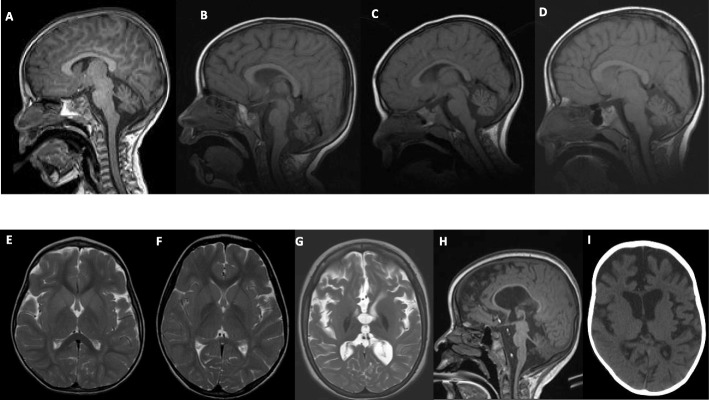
Radiographic Imaging Data for the Study Cohort. a-d: Representative MRI images for the affected individuals P14 (at age 3 years 2 months), P15 (at age 3 years 2 months), P19 (at age 4 years 3 months), and P20 (at age 4 years 7 months) showing cerebellar atrophy. e: MRI at 3 years and 2 months showing altered signal intensity in the globus pallidus of P15. f: MRI at 4 years and 3 months showing bilateral symmetrical subtle low T2 signals noted in the globus pallidus, substantia nigra, and dentate nuclei of P19. g: MRI at 6 years and 9 months showing bilateral globus pallidus low signal intensity likely representing iron deposition in P18. h: MRI at 6 years and 9 months showing brain, cerebellar and brainstem atrophic changes in P18. i: CT at 6 years showing global brain atrophy in P26
